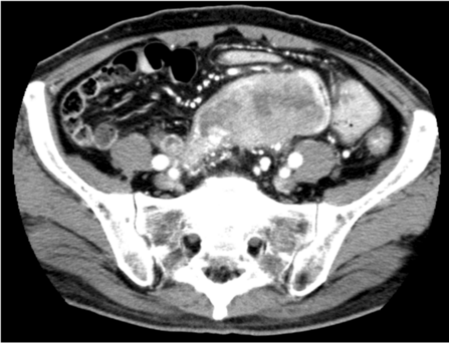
Case 2: abdominal CT scan showed a large mass in the sigmoid colon
